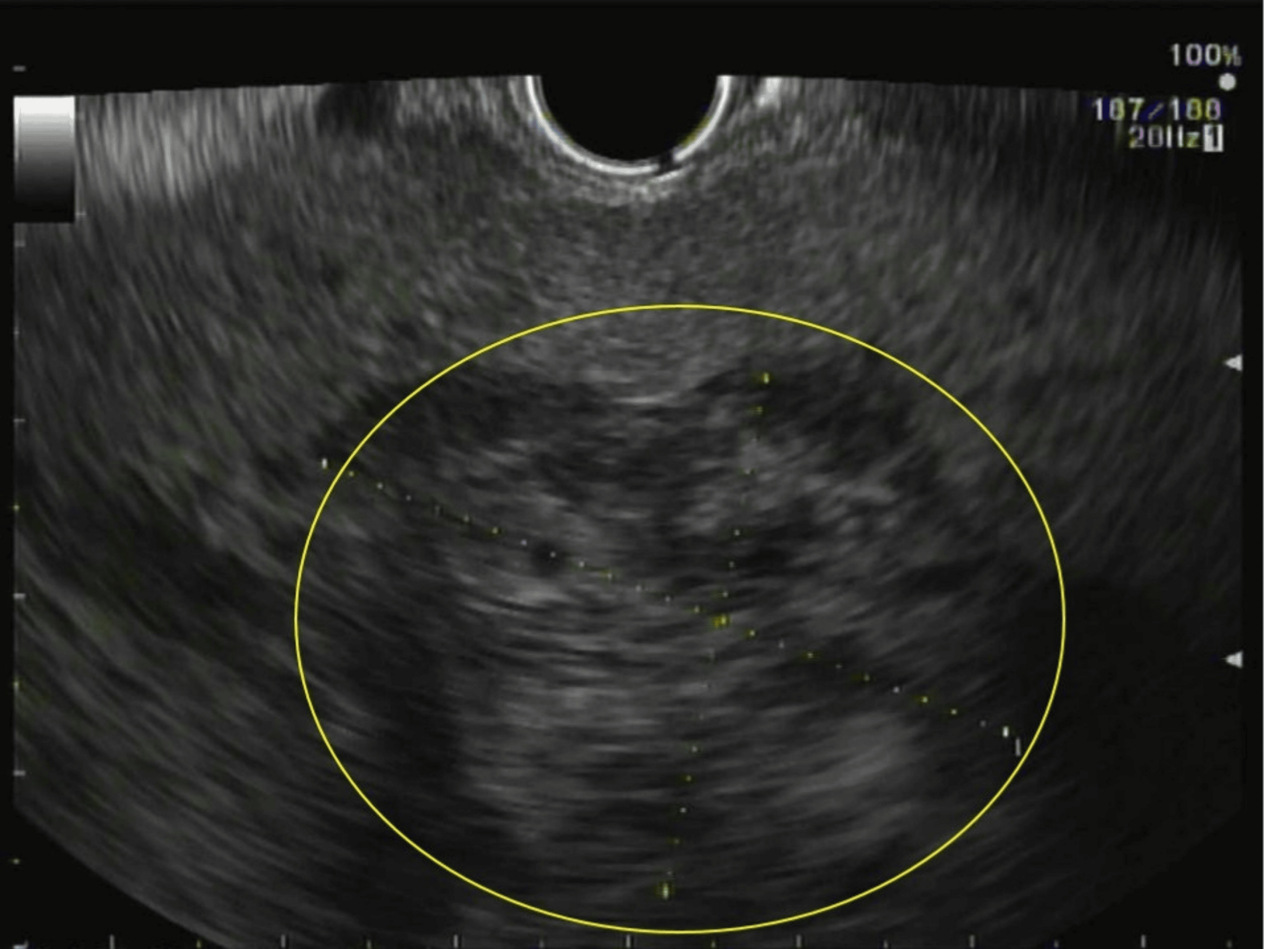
EUS image EUS image obtained with a therapeutic curvilinear echoendoscope demonstrates a large, heterogeneous mass (circled) around the pancreatic head (dimensions 6.9 × 4.1 cm), extending into the hepatic hilum as a contiguous 4.25 × 2.97 cm lesion. The mass involves multiple blood vessels (including the splenic and common hepatic arteries), encases the extrahepatic bile duct, and compresses the main pancreatic duct (measuring approximately 2.7 mm).​ EUS, Endoscopic Ultrasound
